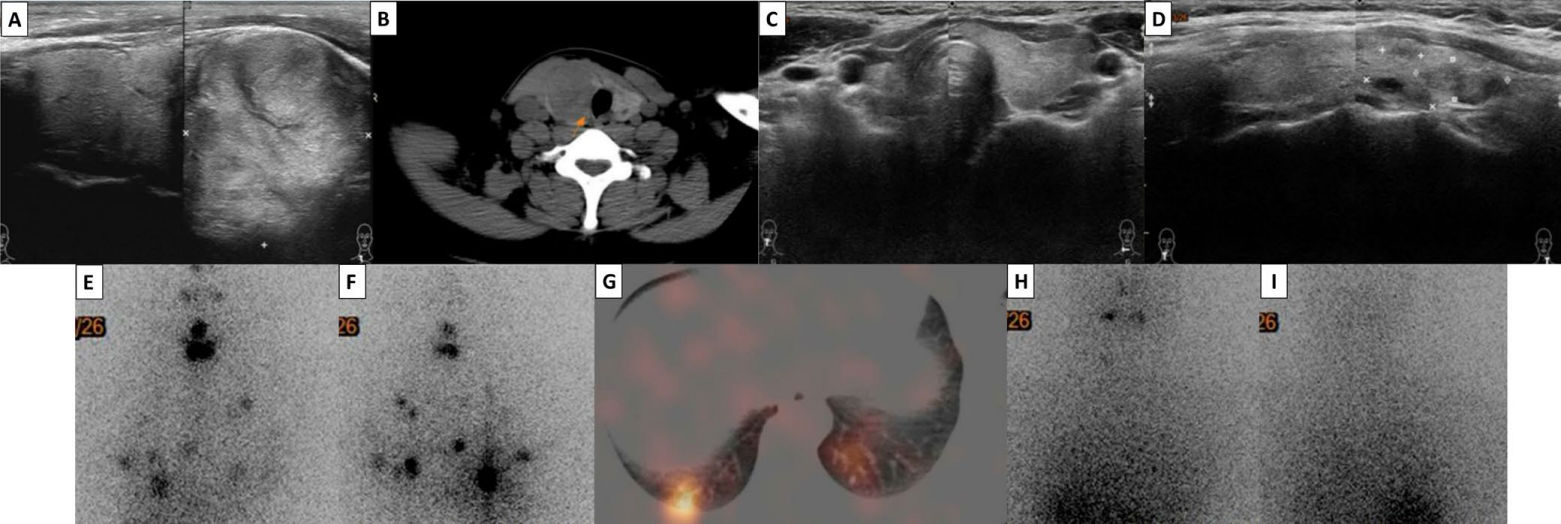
(A–C) An 18‐year‐old female with a large right thyroid nodule (4 cm) underwent right lobectomy in 2012. (D) Follicular thyroid cancer located in the left thyroid lobe was diagnosed in 2019 after several years of neck ultrasonography follow‐up. (E–G) The patient received an RAI dose of 150 mCi after complete thyroidectomy, and multiple pulmonary metastases were observed on post‐RAI imaging. (H and I) An additional RAI dose of 200 mCi was administered, and the post‐treatment scan appeared negative.
